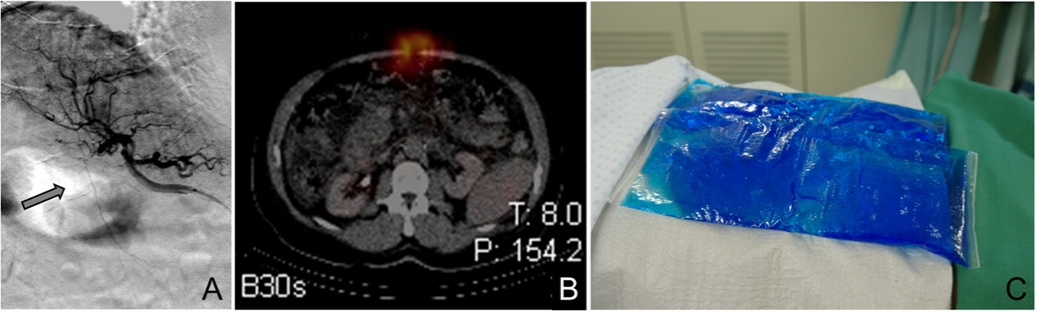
Example of a not coilable hepatic falciform artery (HFA). Patent HFA detectable in DSA (A) which could not be coil embolized. In the SPECT/CT an abdominal wall ^99m^Tc-HSAM acculumulation was detected (B), hence to prevent SIRT sequela ice packs were placed on the abdominal wall prior to 90-yttrium administration (C) which was finally tolerated well.
